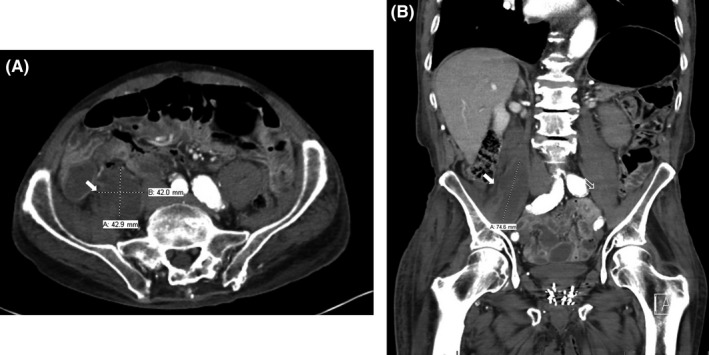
Coronal (A) and axial (B) section of the CT abdomen showing right psoas hematoma (filled arrows) vs normal structures (unfilled arrow)
